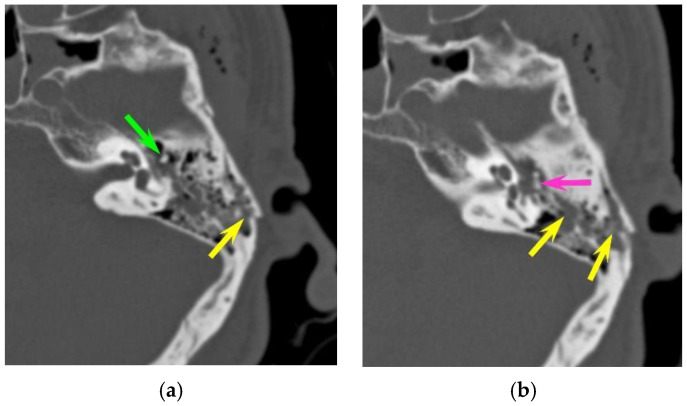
Ossicular chain dislocation. A polytrauma patient with otorrhagia. CT of the left temporal bone (axial plane). (a,b) Longitudinal fracture (yellow arrow); the malleus head (green arrow) is displaced and isolated from the incus body (pink arrow), corresponding to an incudo-malleolar luxation.
